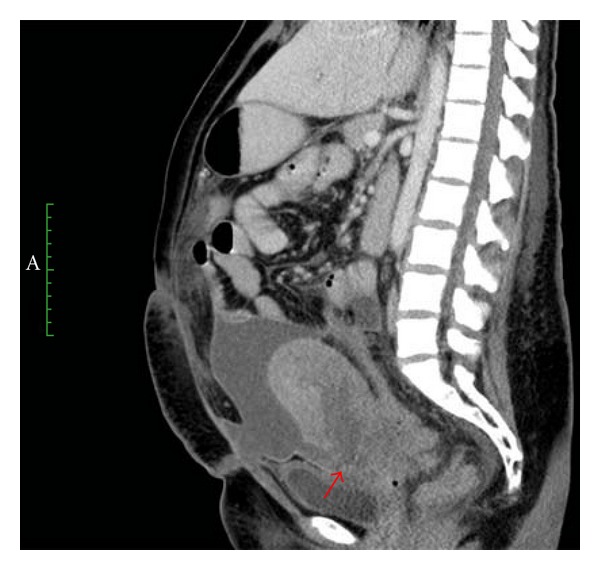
This sagittal CT of the abdomen better demonstrates the dehiscence of the anterior, lower uterus (arrow), and the peritoneal fluid. The endometrial cavity is distended with fluid that is contiguous with the peritoneal space.
